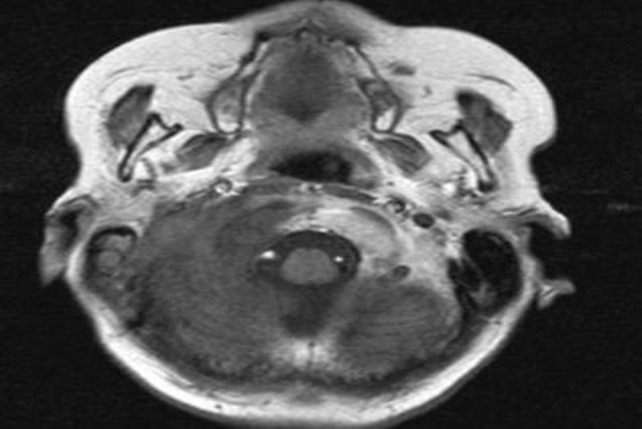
MRI of the brain. Axial T1 weighted sequence demonstrates loss of normal signal intensity over the right mastoid, and the heterogenous lesion in the medial part of the mastoid extends to the petrous apex with involvement of the clivus
